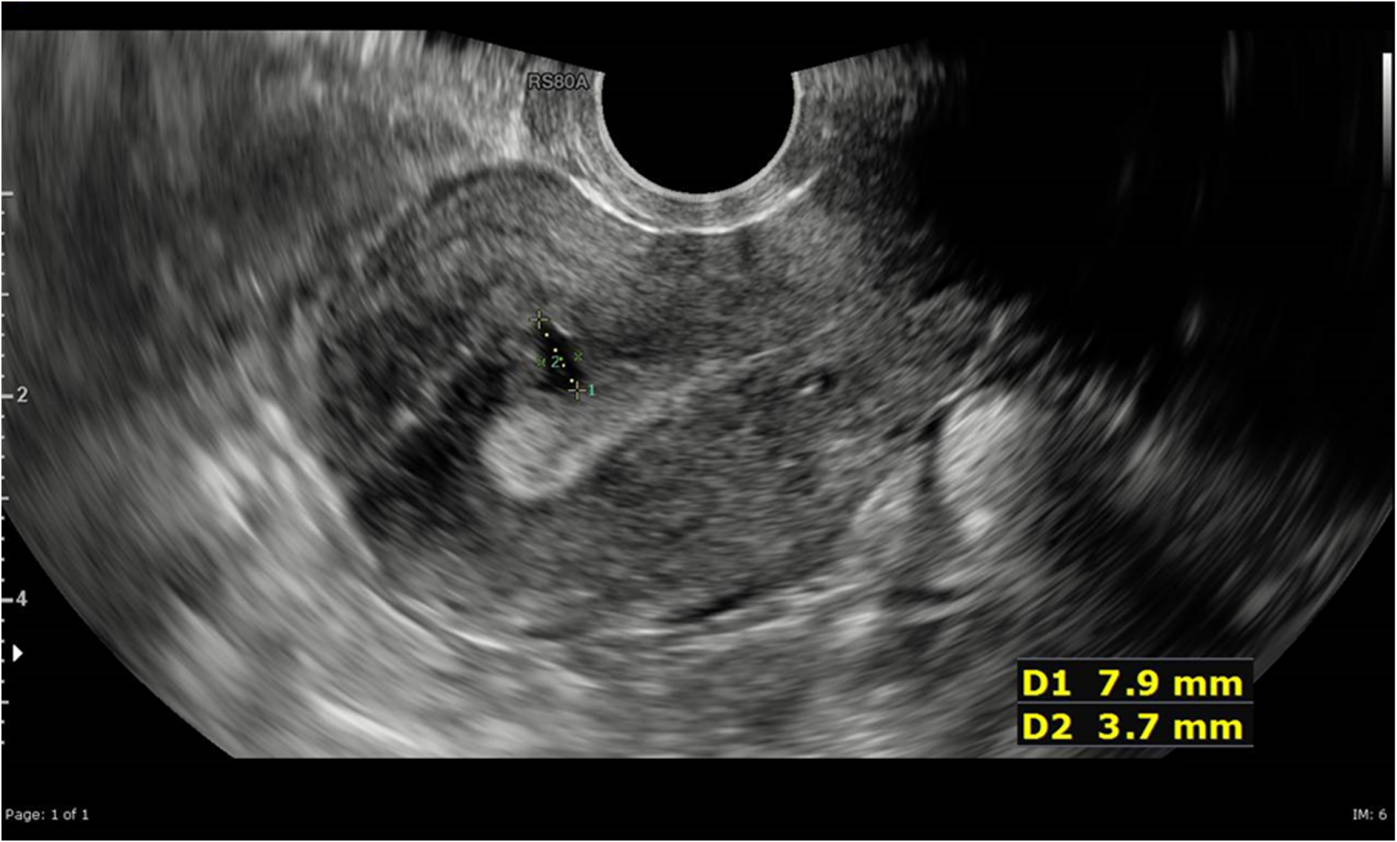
Transvaginal ultrasound showing echotexutre at the fundus
